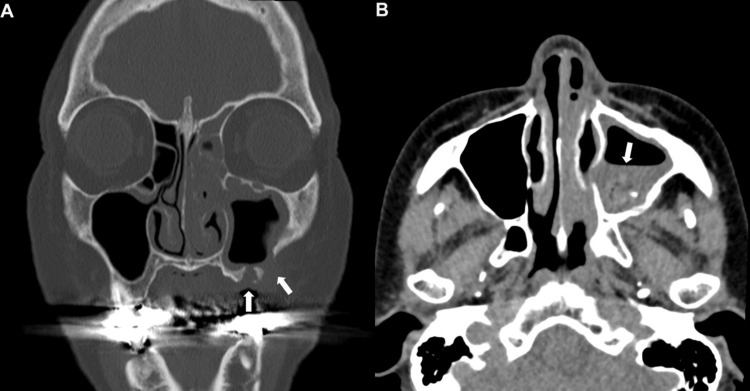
Coronal bone-window CT scan (A) showing mucosal thickening in the left maxillary, ethmoid, and frontal sinuses, with reactive thickening of the left maxillary sinus wall. Changes of post-implant removal and a Caldwell-Luc antrostomy are visible (white arrow), with no bone destruction. Axial soft-tissue window CT scan (B) showing a roundish high-density area with punctate calcifications in the center of the left maxillary sinus (white arrow).
